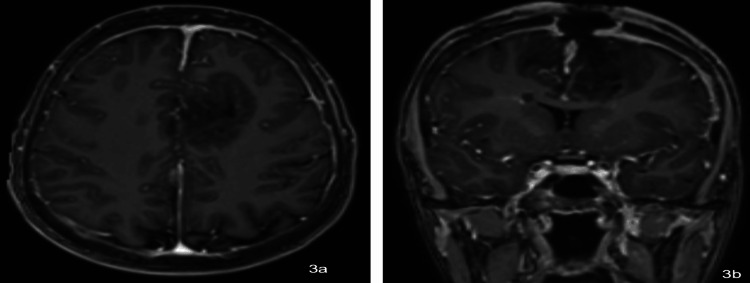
Postoperative day 1 MRI in (a) axial and (b) coronal planes showing slight pneumocephalus and cerebral edema without midline shift
